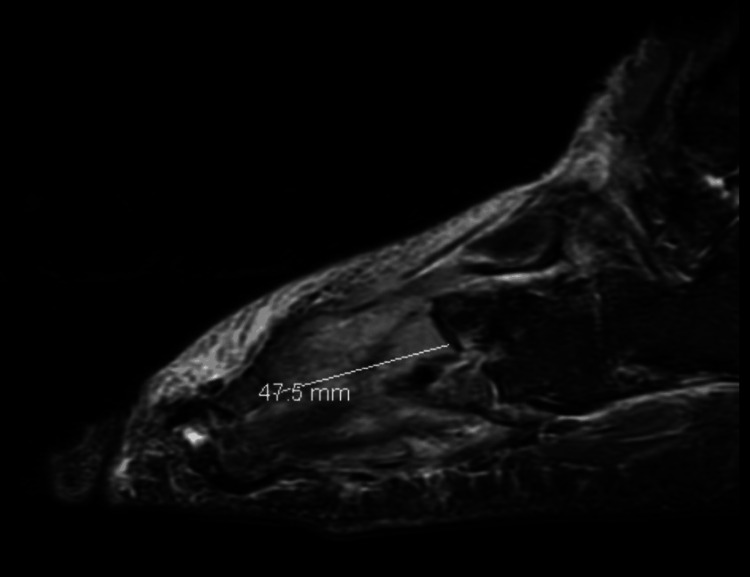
Initial non-contrast foot MRI revealed an ill-defined soft tissue mass involving the base of the third metatarsal, concerning for malignancy or infection. A 4.75 cm ill-defined soft tissue mass was noted involving the base of the third metatarsal. MRI: magnetic resonance imaging
